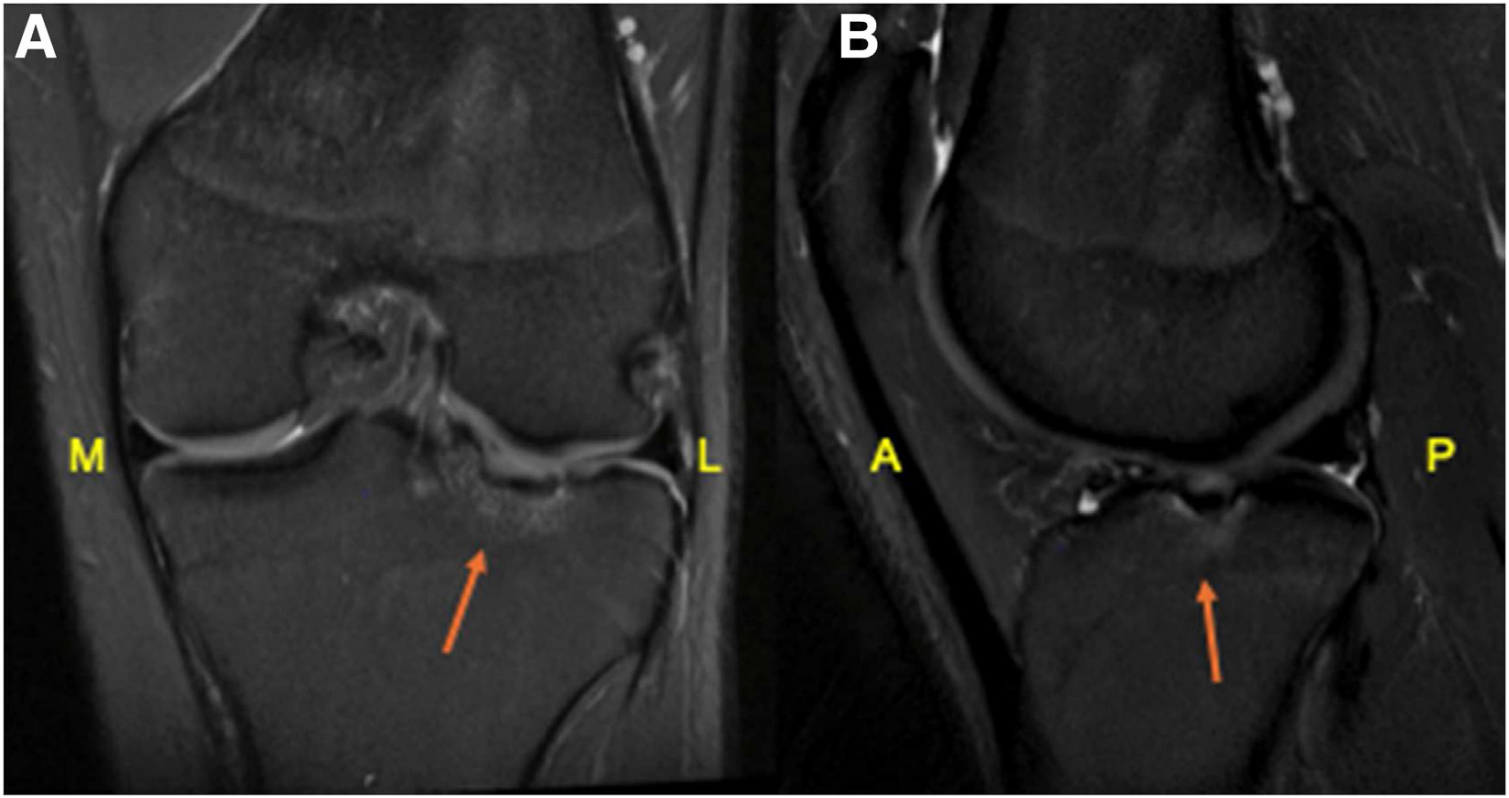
Preoperative magnetic resonance imaging showing an osteochondral defect measuring 16 × 16 × 5 mm in the central aspect of the lateral tibial plateau, shown on coronal (A) and sagittal (B) views. Red arrows indicate the defect location. Yellow labels denote anatomic orientation. (A, anterior; L, lateral; M, medial; P, posterior.)
